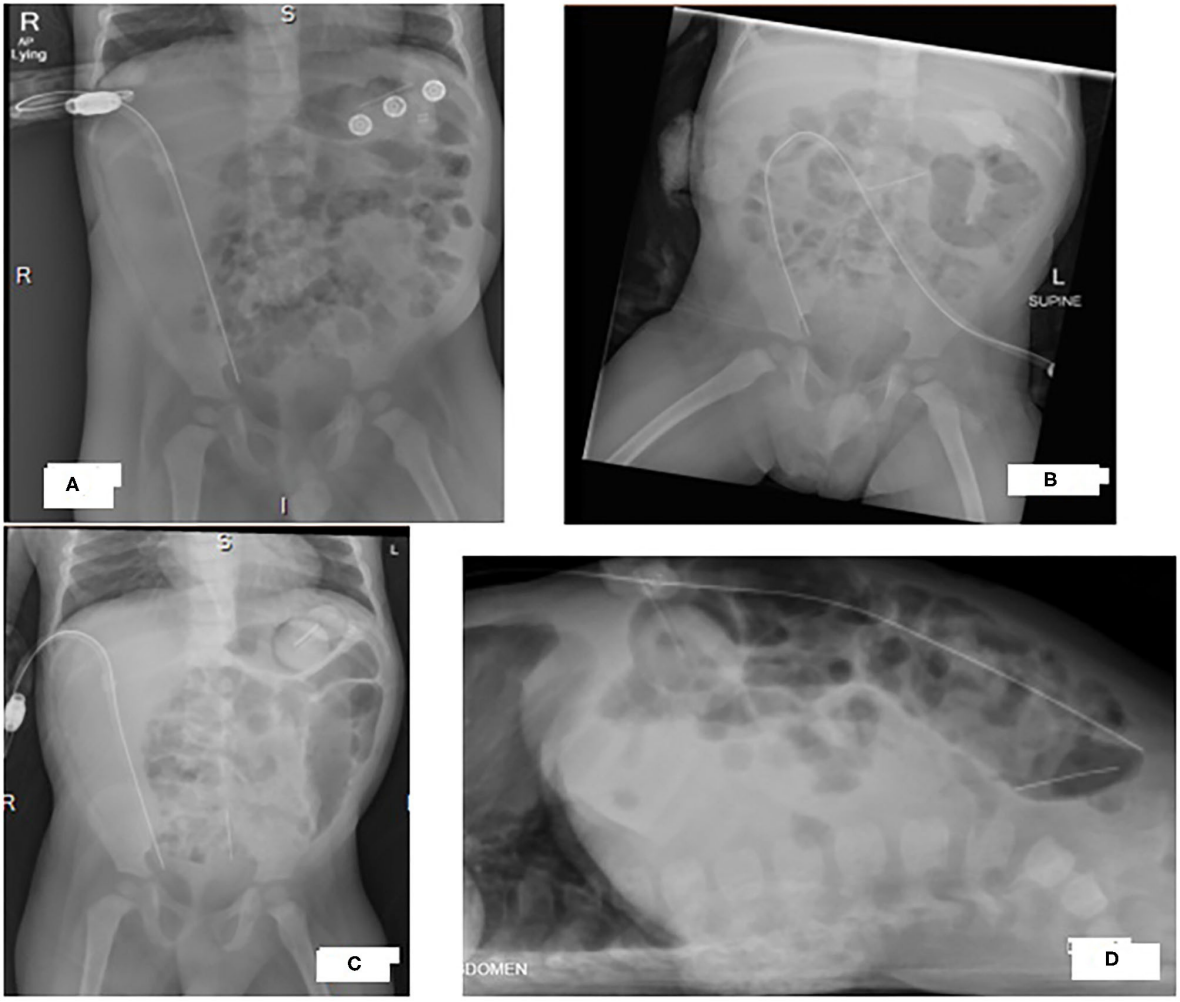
X-rays of our patient's abdomen showing progression of a radio-opaque horizontal thin object from the upper abdomen to the pelvis. (A) Antero-posterior view at 20-month, (B) Antero-posterior view at 22-month (C) Antero-posterior view and (D) cross table view at 24-month.
